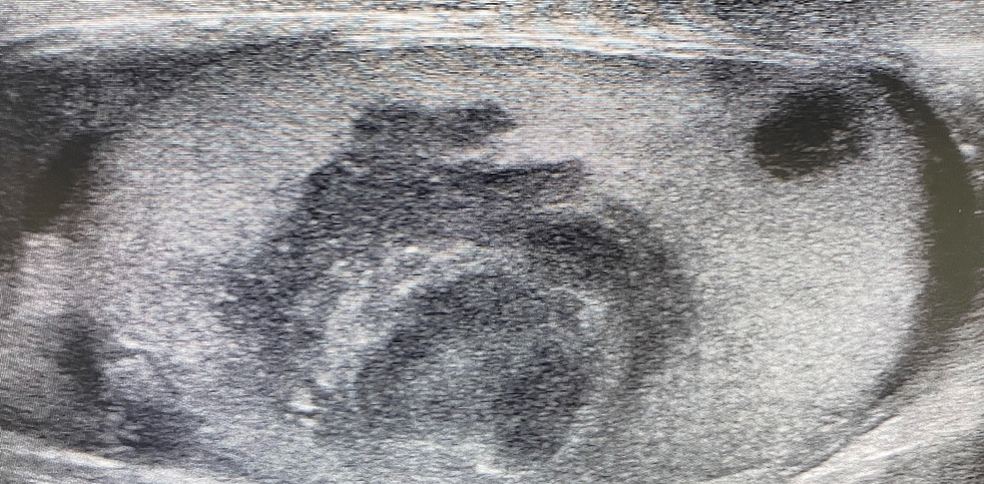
Scrotal sonography at hospitalization Right testis with hypoechogenic mass (up to 30 mm in diameter) in the middle third and smaller hypoechogenic lesion at the lower pole
